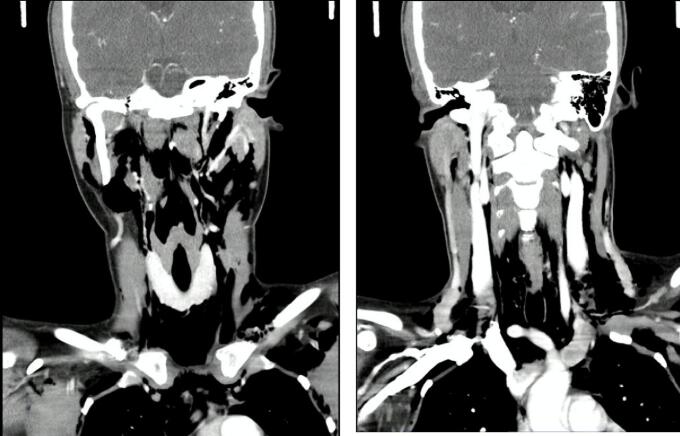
Neck CT scan. Subcutaneous emphysema through the dangerous space.
